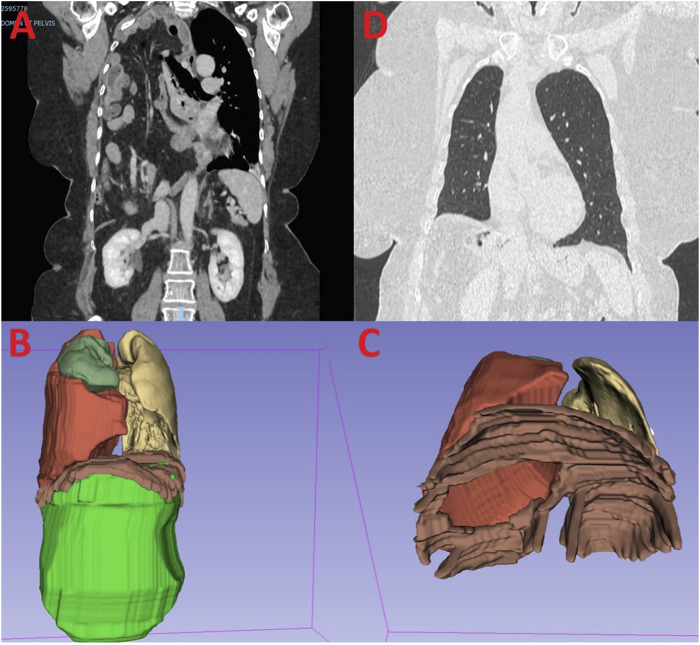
Legend (A) Preoperative CT scan showing a giant diaphragmatic hernia. (B) 3D reconstruction volumetry with the abdominal volume (green), the hernia volume (red), the right lung (yellow) and the left lung (dark green). (C) 3D reconstruction with an inferior view showing the defect through the diaphragm. (D) Post-operative CT scan showing no recurrence at 24 months.
